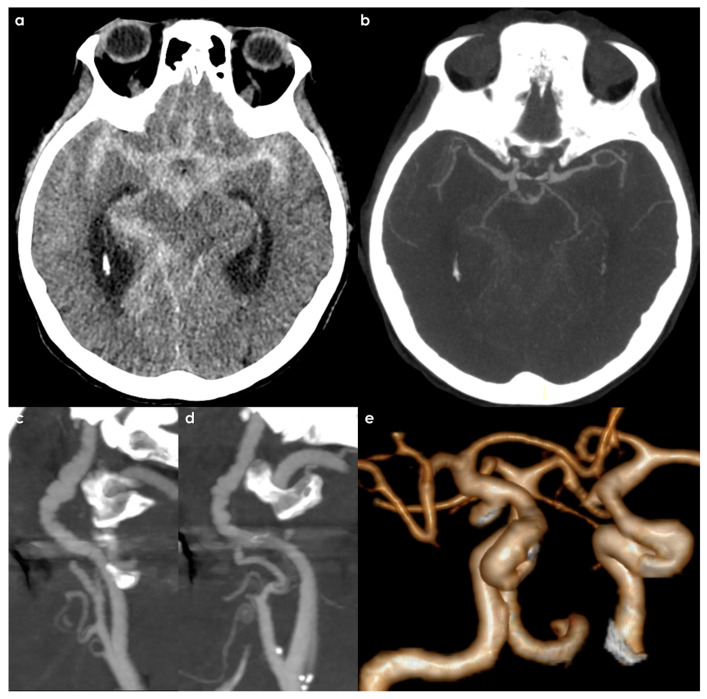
CT and CTA of a patient admitted for the abrupt onset of (thunderclap) headache and progressive decrease in consciousness. The non-contrast brain CT (panel (a)) showed a massive SAH (Fisher grade 4). The CTA (panel (b)) highlighted the presence of a right ICA saccular aneurysm in the communicating segment (MIP/MPR images). In the same investigation, both right (panel (c)) and left (panel (d)) ICA have a multifocal FMD pattern. A volume rendering reconstruction of the intracranial arteries (panel (e)) gives more details about the morphology and surface of the aneurysm.
